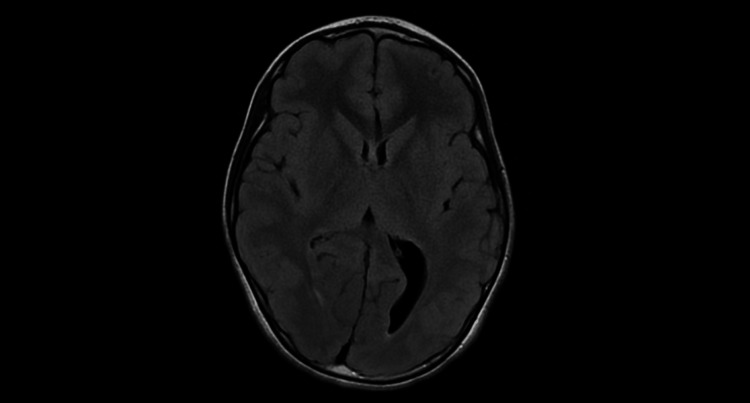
Brain MRI showing asymmetric ventriculi. MRI: magnetic resonance imaging
